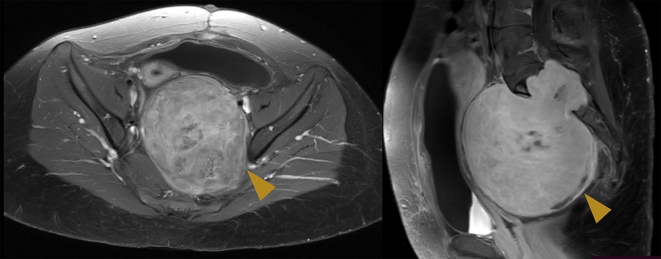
Magnetic resonance imaging after pregnancy. On the left, an axial scan showing the same retroperitoneal Schwannoma (arrowhead); on the right, a sagittal scan with the retroperitoneal Schwannoma (arrowhead).
